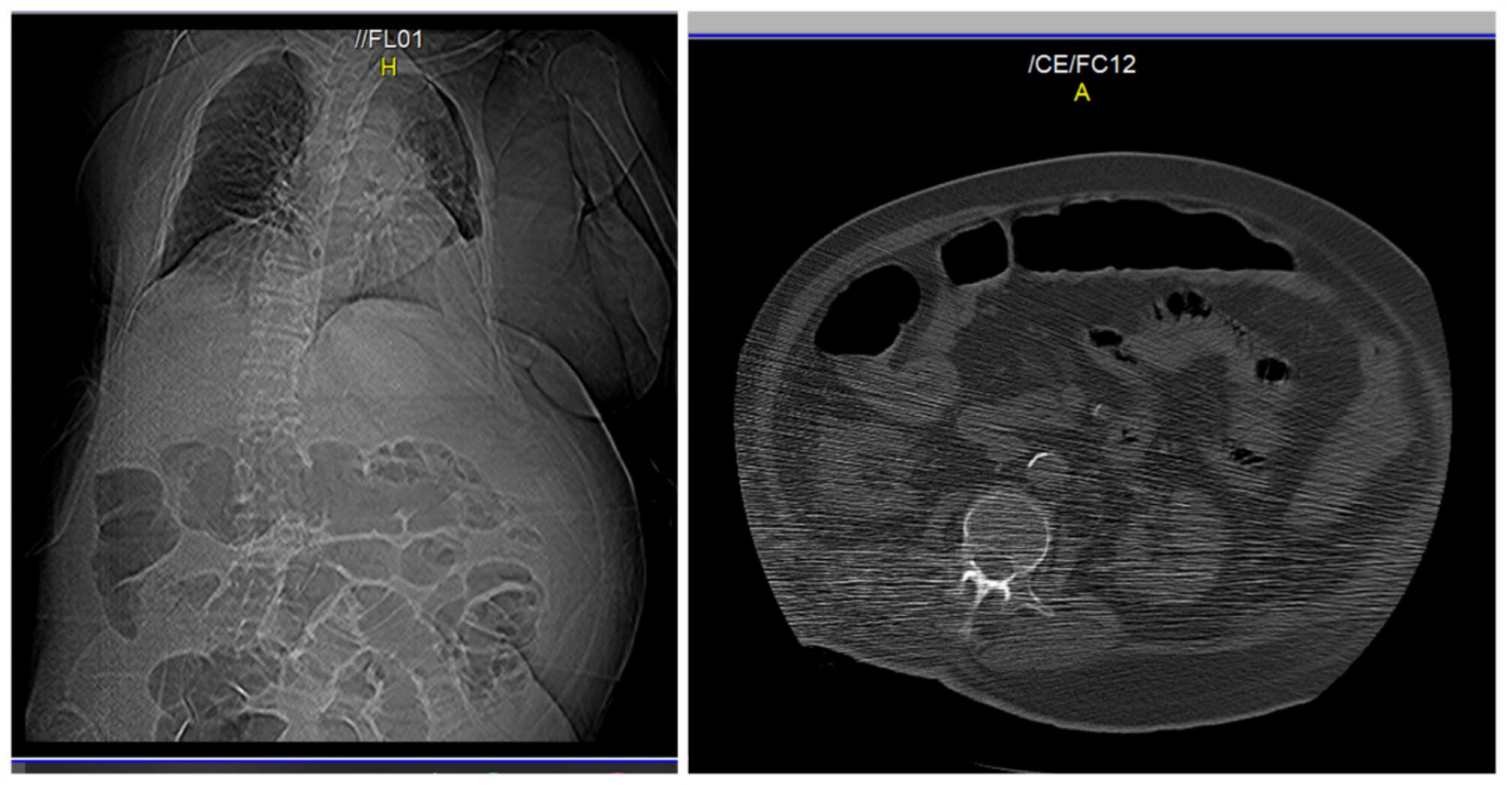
New abdominal CT after the management plan showed decrease in colon diameter
